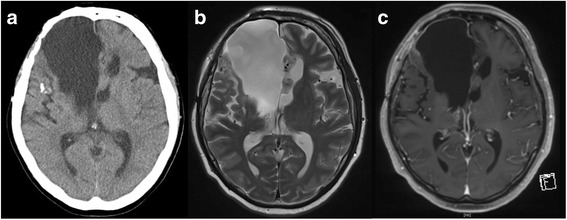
a Brain computed tomography showing a surgical cavity following resection of the tumor in the right frontal lobe and calcification. Magnetic resonance imaging showing the same cavity on a T2-weighted axial image (b) and no ring-enhancing lesions suggesting a brain abscess on a gadolinium-enhanced, T1-weighted axial image (c)
